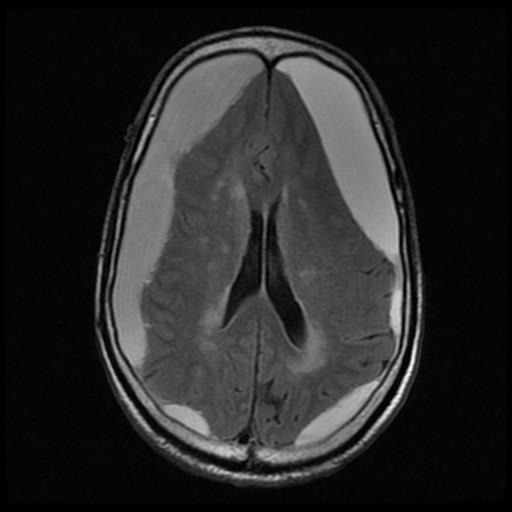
Bilateral chronic subdural haematoma; what should be taken into account is the absence of the mass effect on the structures of the median line, and the presence of the compressive effect on the noble brain structures – MRI image, T1
